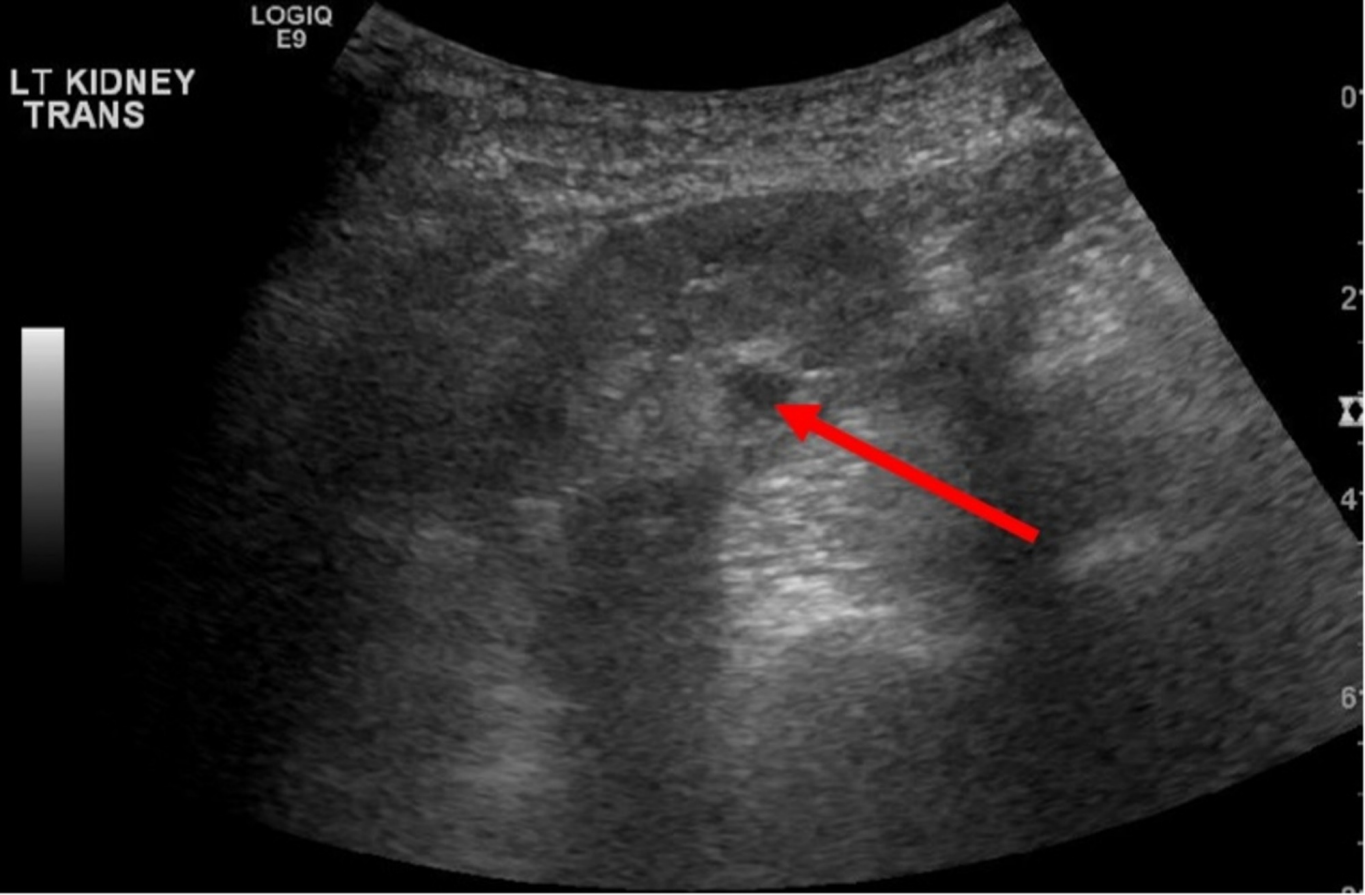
Ultrasound of left kidney. Arrow indicates 1.2 x 0.9 x 1.0 cm cyst in mid-pole region.
